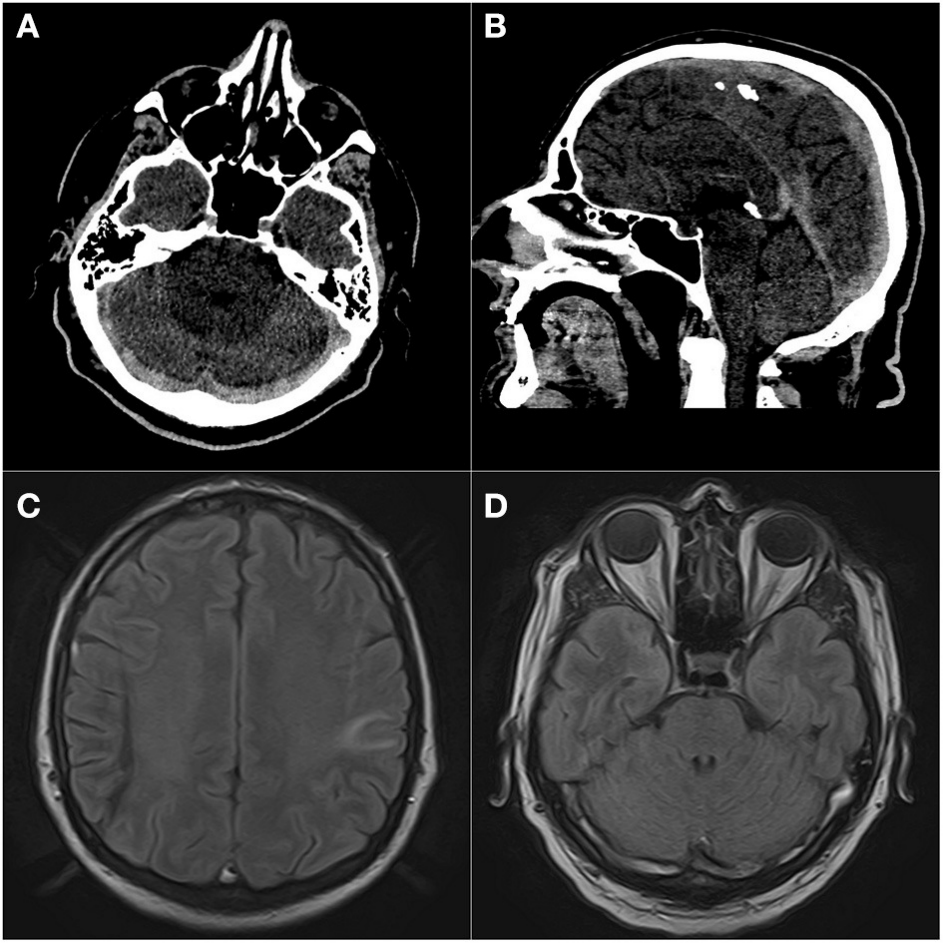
Massive dural sinus thrombosis. Prominent hyperattenuation of transverse (A), superior sagittal, and straight sinuses (B) on non-contrast CT. FLAIR images (C,D) of the same patient show abnormal high signal in dural sinuses and hyperintense area in the left parietal lobe consistent with venous infarct. Patient presented with impaired consciousness.
